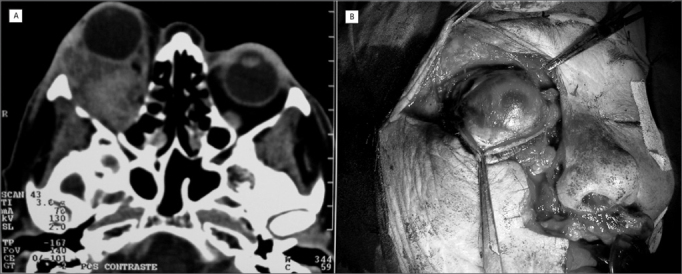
Photography showing an orbit primary melanoma in a CT Scan axial view (A) and during exenteration of the right orbit via broadened Weber-Fergusson incision towards the eyebrow region (B).
